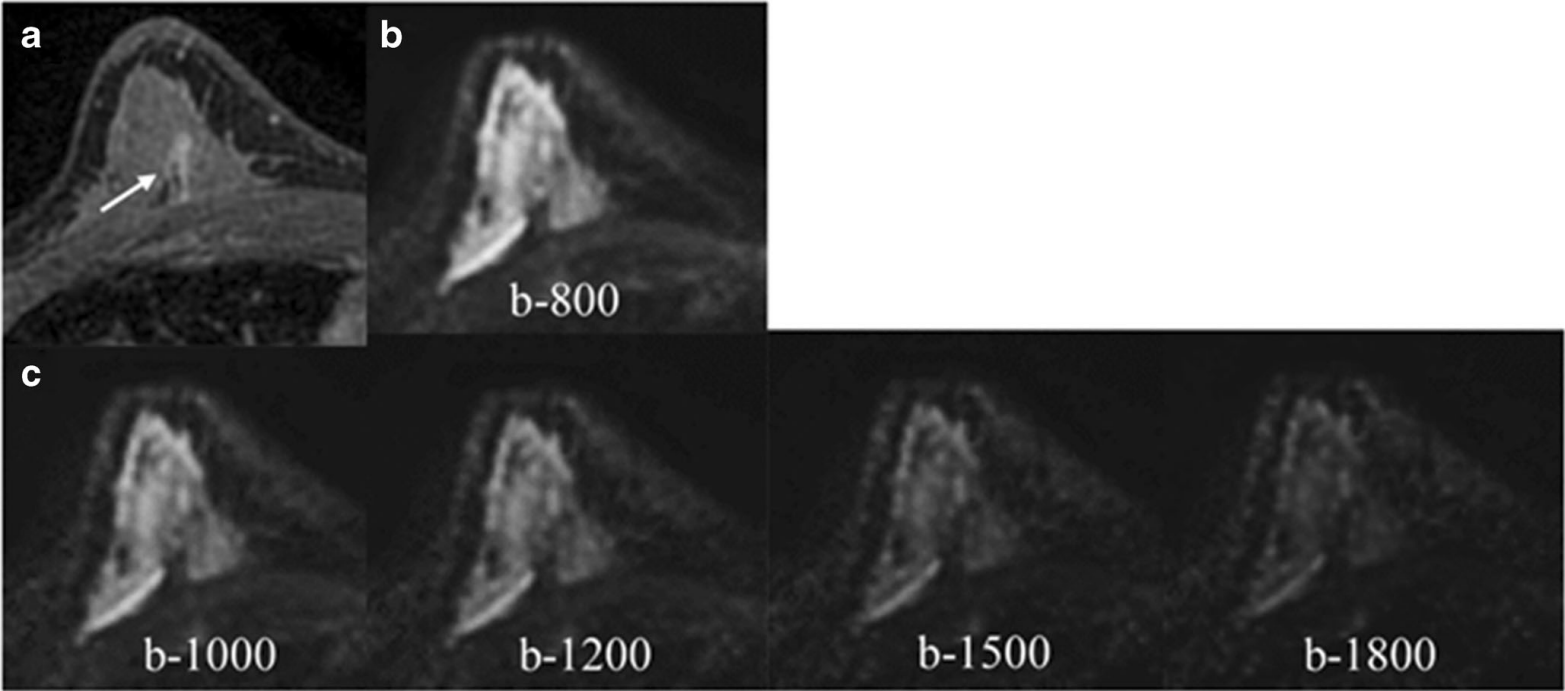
Axial images of a biopsy-proven DCIS in a patient with dense breast. Contrast-enhanced image (a) shows a 14-mm non-mass enhancement in the posterior third of the right breast (arrow) in the middle third. All readers missed this lesion on DWI which was indistinguishable either in the acquire DWI b-800 (b) or the synthetic b-values (c). DCIS, ductal carcinoma in situ; DWI, diffusion-weighted imaging
